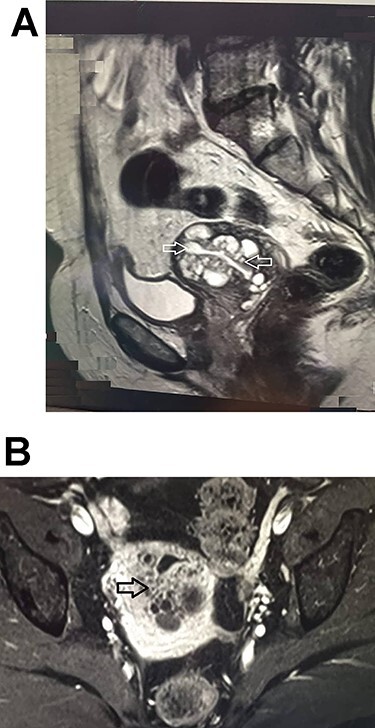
MRI appearance of pelvic before surgery with contrast. There are multiple lesions invading from the endocervical glands to cervical stroma and solid components (A). Sagittal T2-weighted image shows the cervical tumor (arrows). (B) Axial T1-weighted image of the cervix with irregular multicystic lesions suggesting MDA (arrow).
